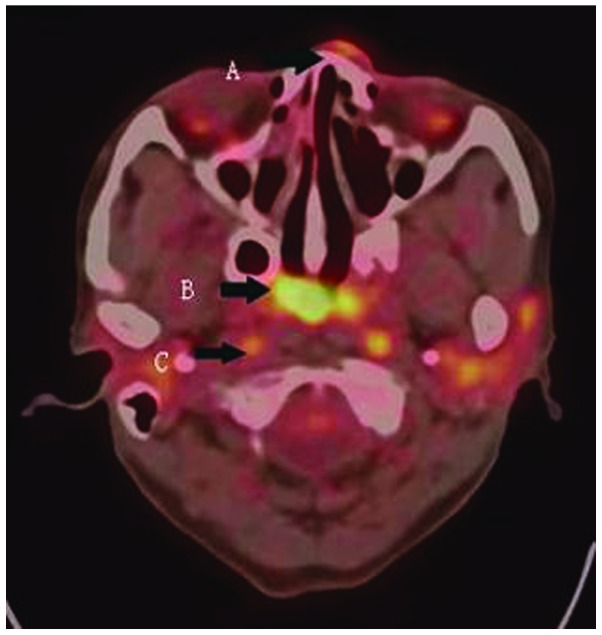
^18^F-FDG uptake in the lesions detected by positron emission tomography/computed tomography. The (A) left nasal alar lesion, (B) nasopharyngeal neoplasm and (C) parapharyngeal lymph nodes demonstrate ^18^F-FDG uptake. ^18^F-FDG, 8-fluorine-2-fluoro-2-deoxy-*d*-glucose.
